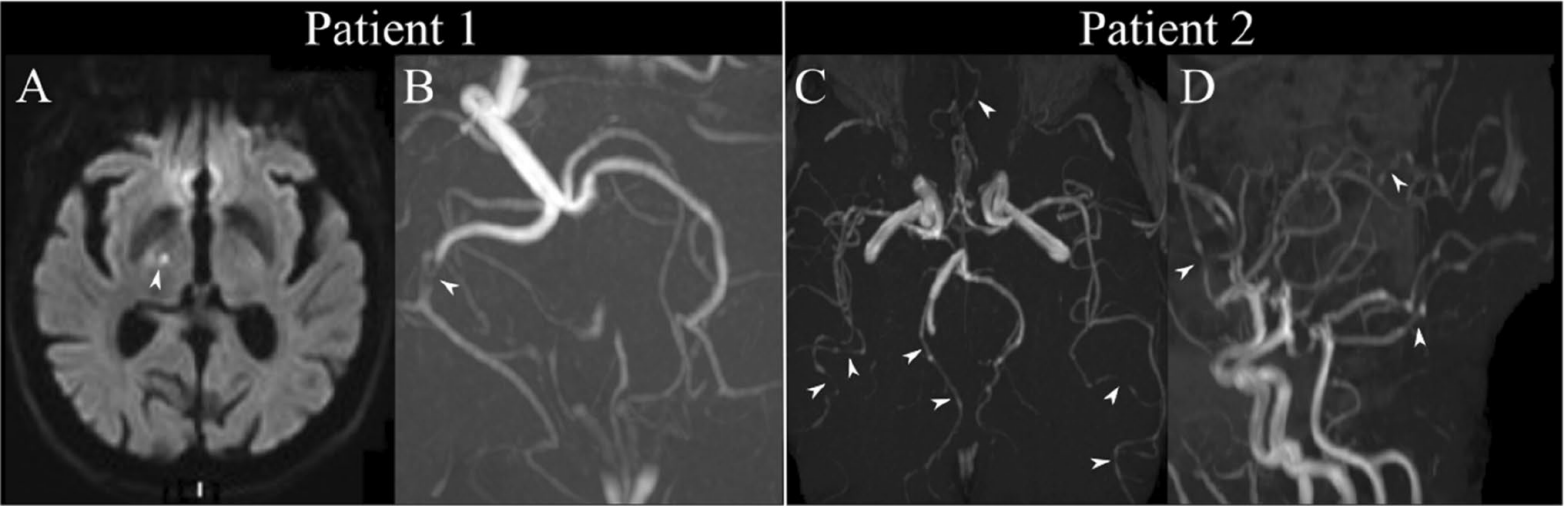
Patient 1 (left): A hyperintensity in the right ventro-lateral thalamus on the diffusion weighted imaging (DWI) sequence corresponding to a punctiform acute ischemic lesion and B significant stenosis of the P2 segment of the right posterior cerebral artery on the time-of-flight (TOF) sequence (white arrow). Patient 2 (right): C, D multiple arterial irregularities (white arrows) on the TOF sequence suggestive of reversible cerebral vasoconstriction syndrome
